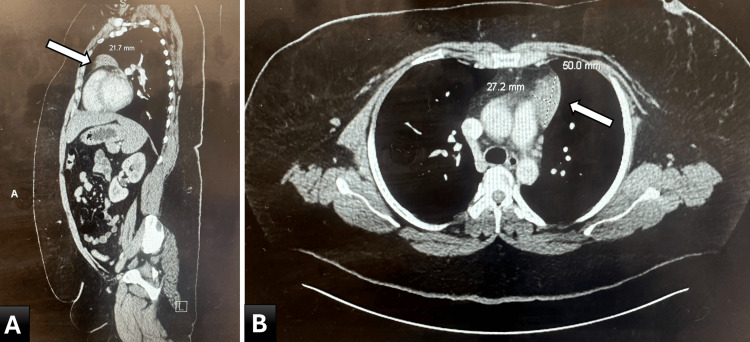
CT demonstrating anterior mediastinal mass. (A) Sagittal and (B) axial contrast-enhanced CT images of the chest demonstrating a well-defined anterior mediastinal mass (arrows). These findings are characteristic of mediastinal involvement in T-lymphoblastic leukemia/lymphoma.
